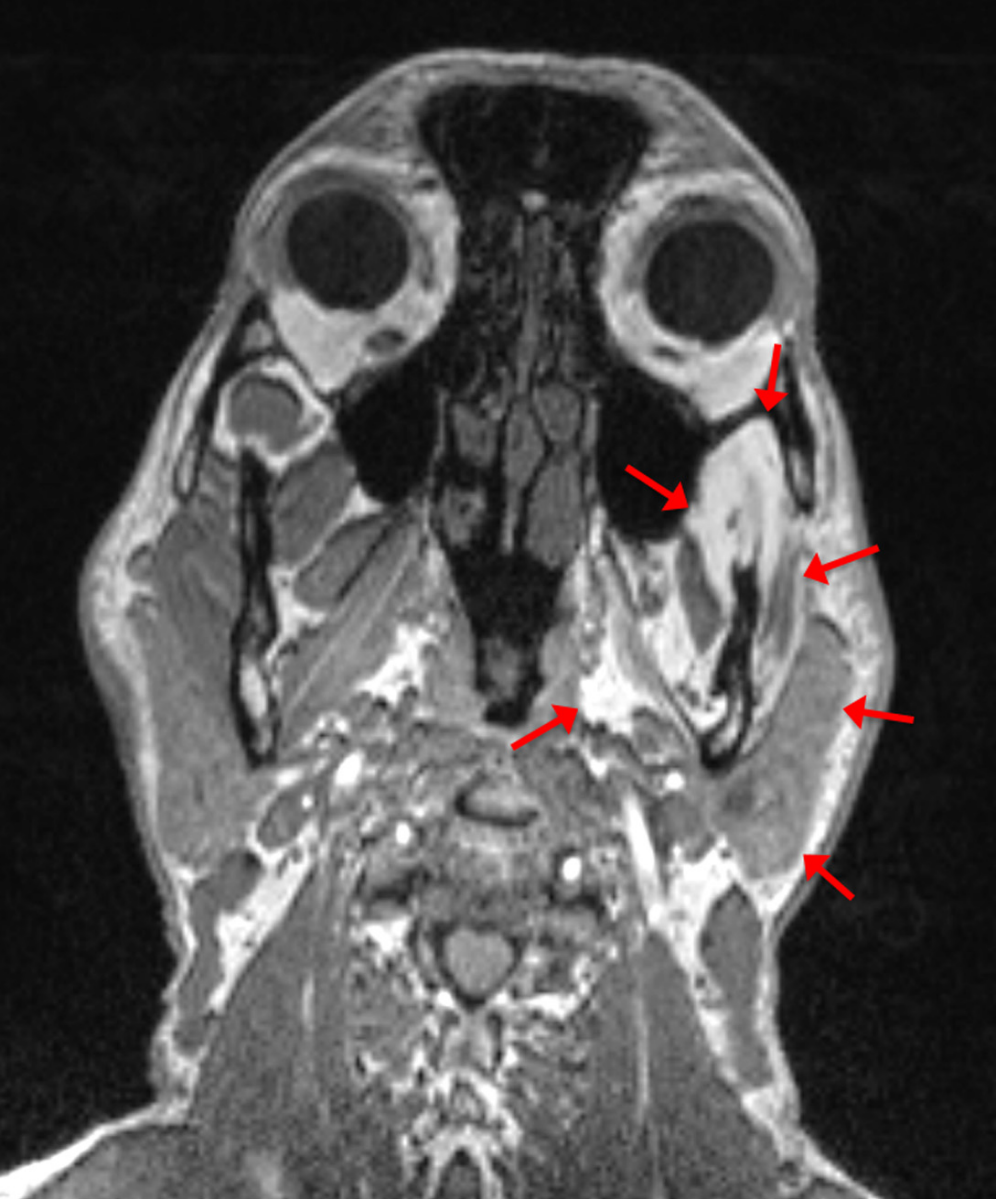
According to MRI scanning, the length of the condylar‐angulus measure asymmetry was 4 mm. Measurements were recorded from axial T1 weighted and coronal T2 weighted images. Muscle tissue replacement with fat tissue and atrophy of masticatory muscles were also seen in MRI scanning (red arrows).
